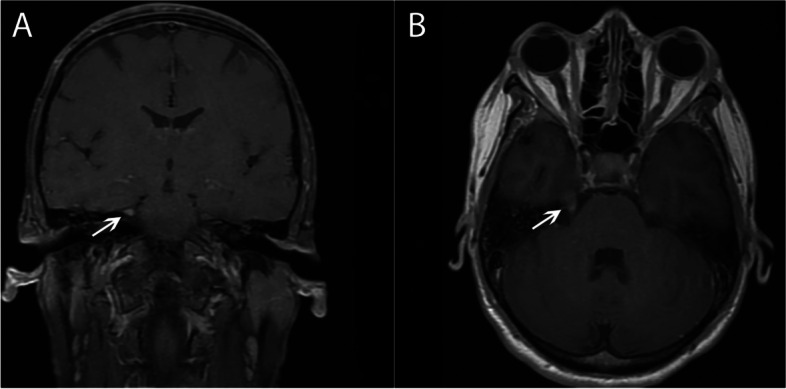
T1-post contrast coronal (A) and axial (B) MRI demonstrating bilateral (right greater than left) cranial nerve V enhancement
